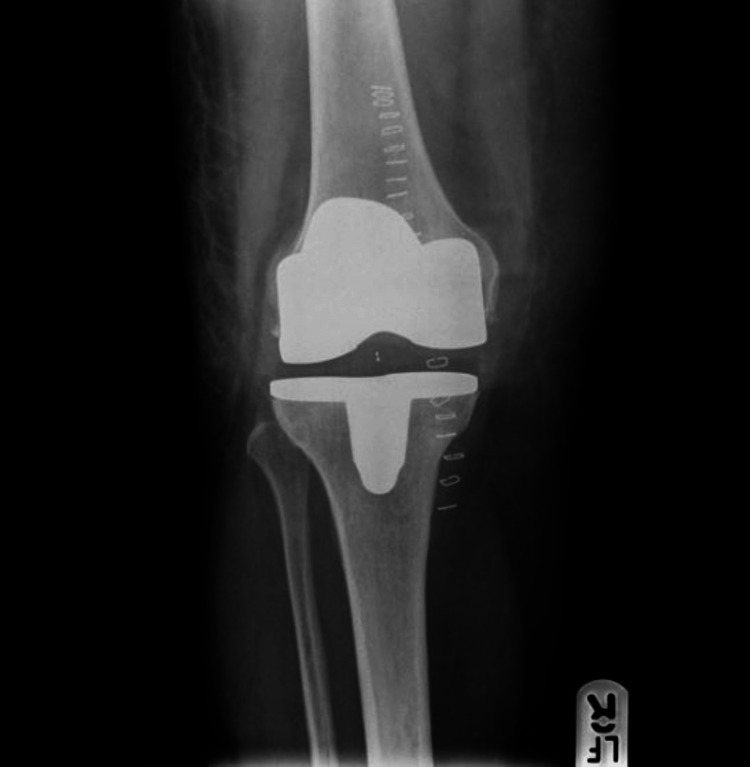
Postoperative X-ray, AP view AP: anteroposterior
